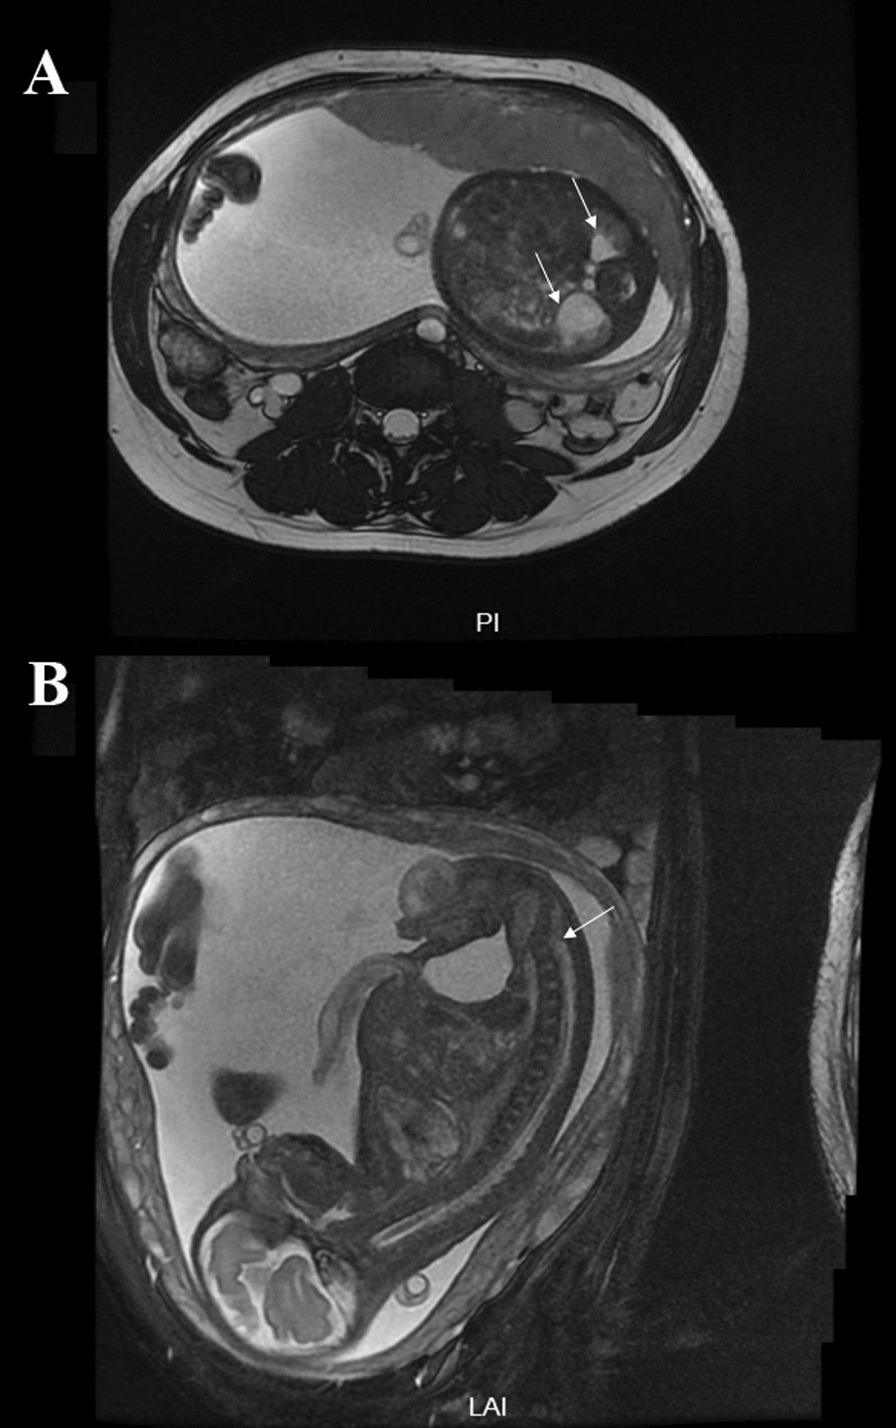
Magnetic resonance imaging. The fetus showed A hydronephrosis, B irregular spine in sagittal section
